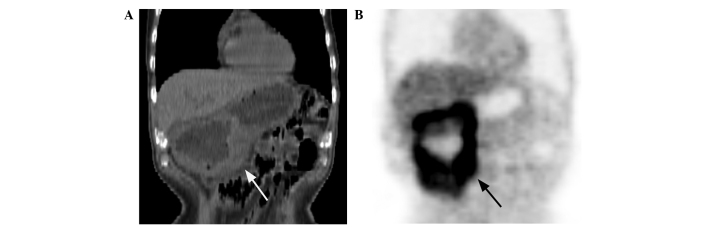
A 78-year-old male with gastric diffuse large B-cell lymphoma. (A) In the coronal computed tomography image, a diffuse thickened gastric wall can be observed in the body and the antrum of the stomach. (B) In the coronal positron emission tomography image, diffuse increased ^18^F-fluorodeoxyglucose accumulation (maximal standard uptake value, 11.3) can be confirmed in the corresponding gastric regions.
